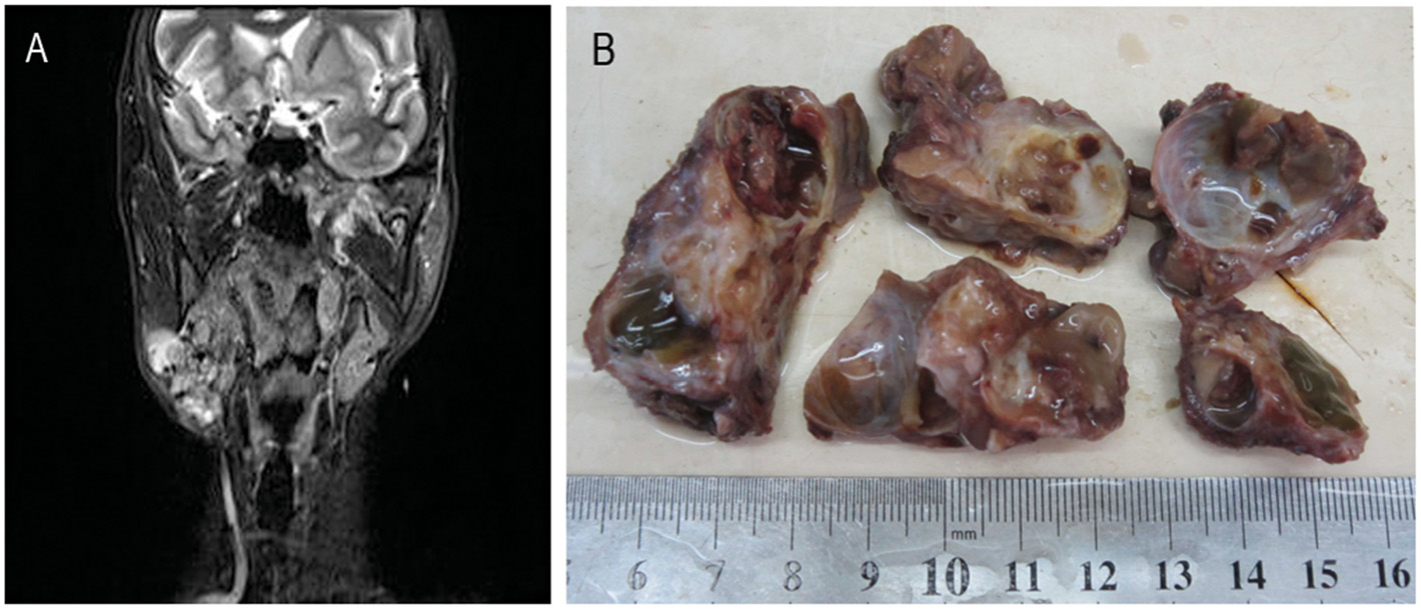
Radiographic examination and gross appearance of the submandibular gland cystadenocarcinoma. (A) MRI scan revealed an irregular mass in the right submandibular gland with multiple cystic regions. (B) The cut surface of mass exhibited a nodular structure with multiple cysts filled with mucus-like materials.
